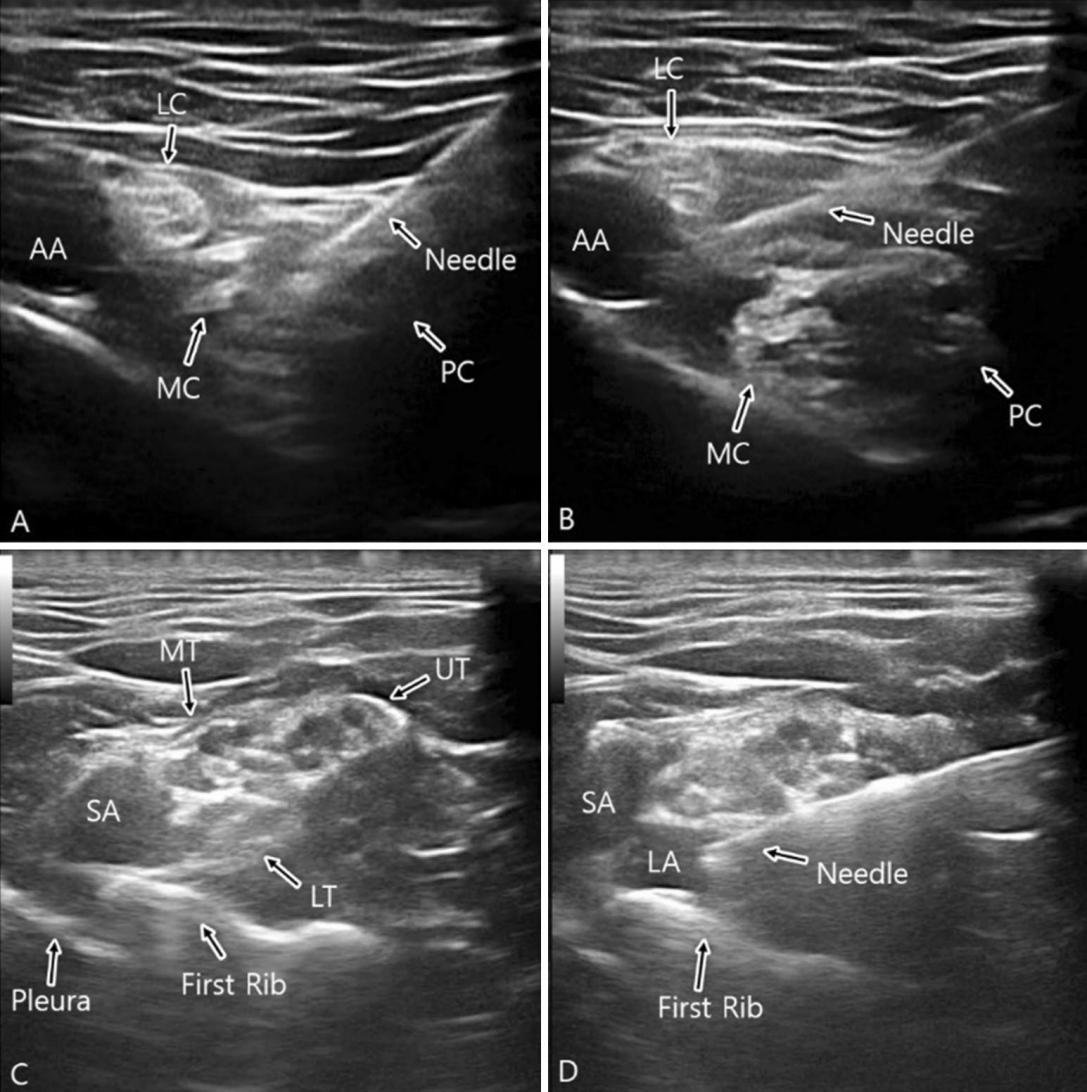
Sonographic view of costoclavicular (A,B) and supraclavicular (C,D) block.
